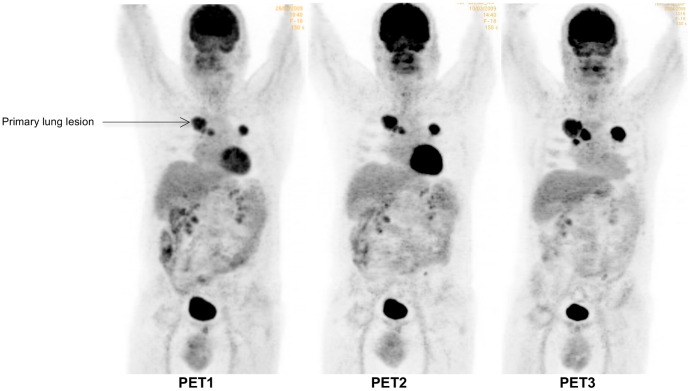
Example of a progressive patient on PET (mP) and conventional imaging. Progressive patient with right upper lobe NSCLC associated with médiastinal lymphadenopathy, lung and bone metastases (patient #2). Sum of the SUVmax of the 5 most hypermetabolic lesions (2 lung lesions, 2 mediastinal lymph nodes, one hilar lesion) were 35.2, 44.3 (+26%) and 59.9 (+70%) for PET1, PET2 (% versus PET1) and PET3 (% versus PET1), respectively. Based on a SUVmax cut-off value of −21.6, the patient was classified as mP on PET2, in accordance with RECIST evaluation on CT scan (performed 57 days after starting erlotinib). mP was confirmed on PET3 with the appearance of a new lesion (subcarinal adenopathy) and a 70% increase of SUVmax.
